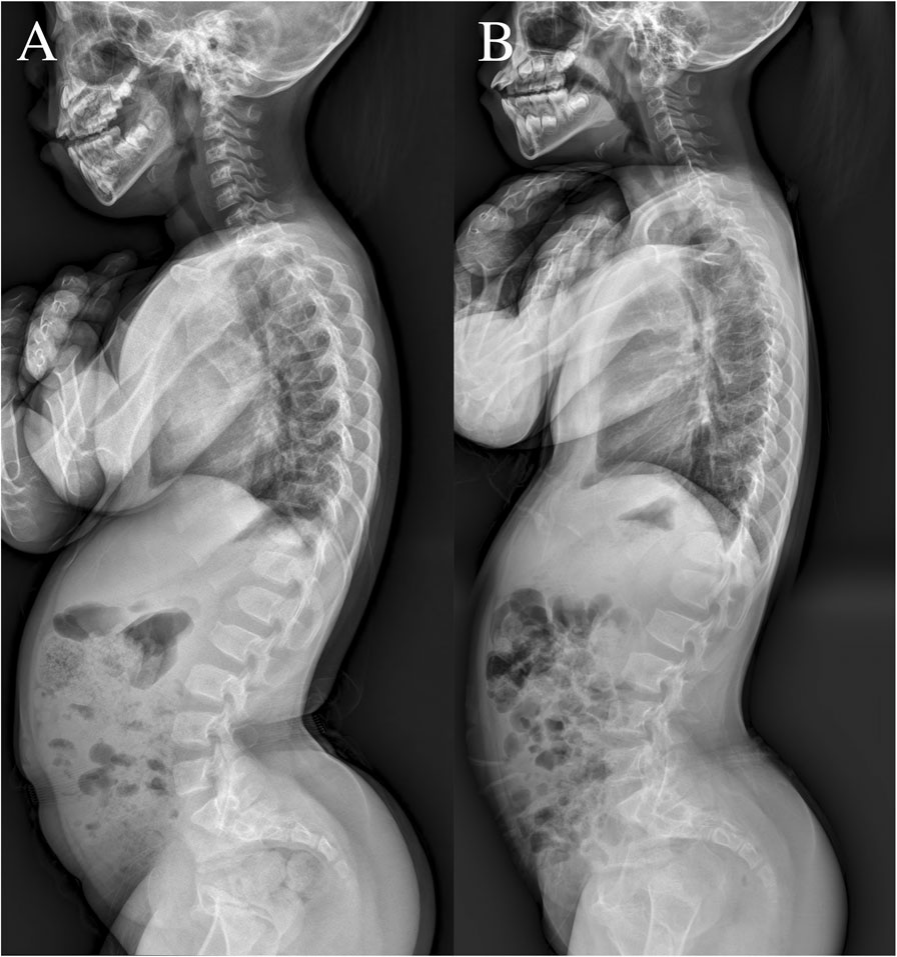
Radiographs of a 16-year-old achondroplasia patient not exhibiting flexion contracture after femoral lengthening. a Initial radiograph of the patient. The patient underwent simultaneous bilateral femoral lengthening to gain 6 cm (lengthening percentage of 19.0%). b Postoperative radiograph showing no significant changes of spinopelvic parameters in comparison with initial radiograph
